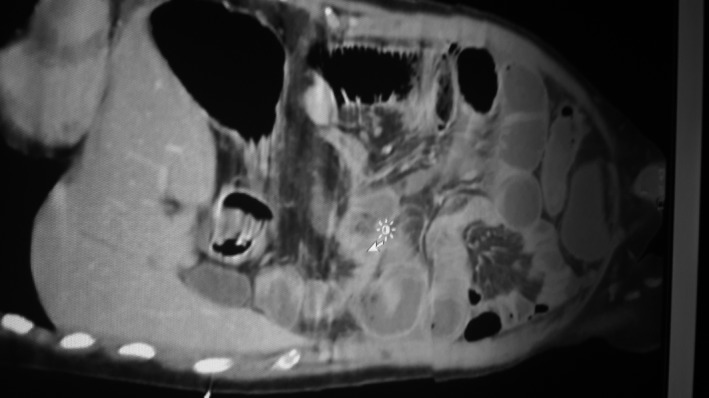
CT Scan abdomen with features of intestinal obstruction.
